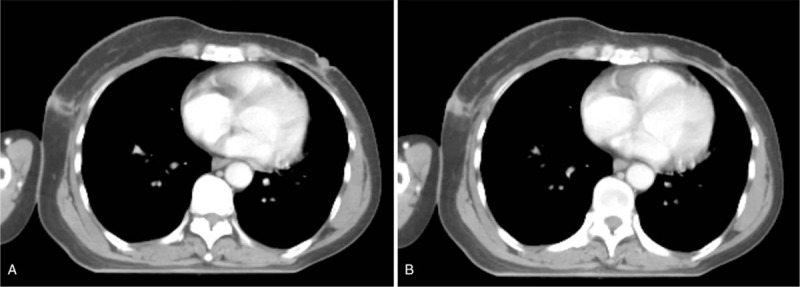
Computed tomographic findings. A,B Computed tomographic images showing the tumor recurred in the scar on the right chest wall.
